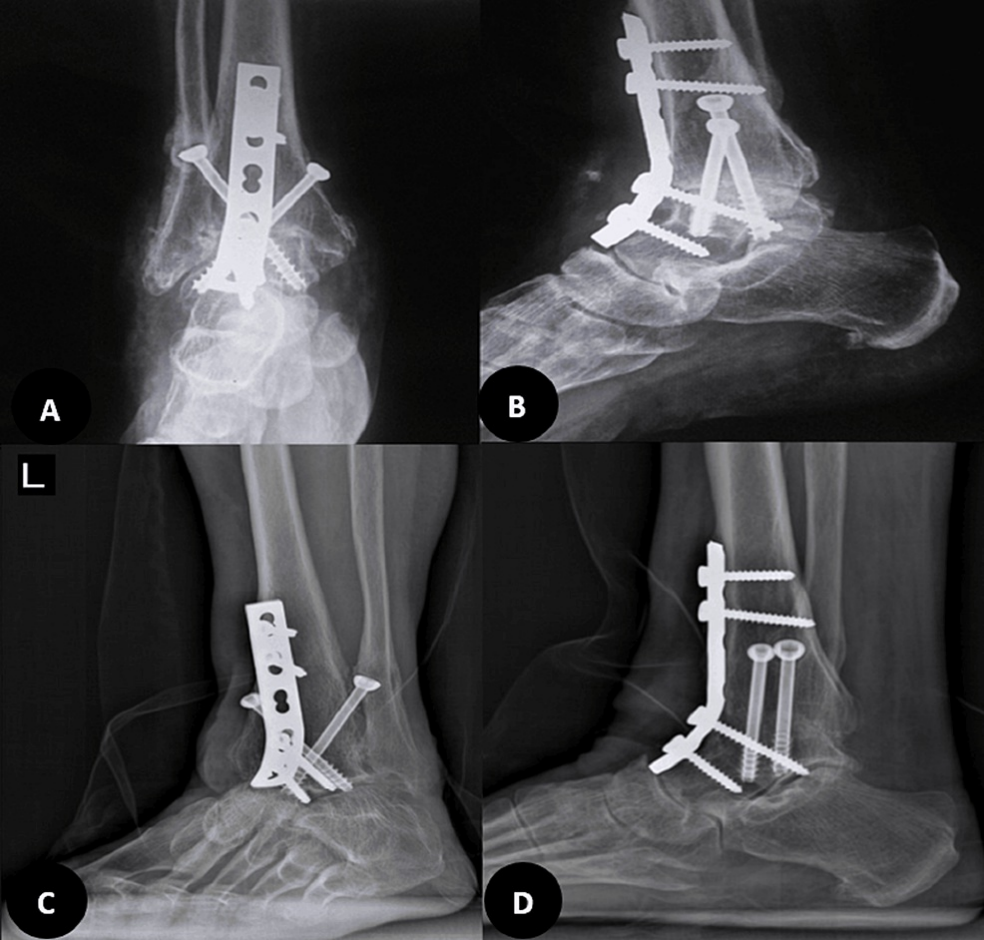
Post-operative radiographies Six months after the ankle surgery, a huge osteonecrosis and fibular stress fracture were managed with an acceptable, painless ankle fusion (not solid) despite the remaining necrosis (A and B, respectively). In 2024, four years after the tumor resection, complete healing of talus necrosis and solid fusion were achieved (C and D, respectively).
